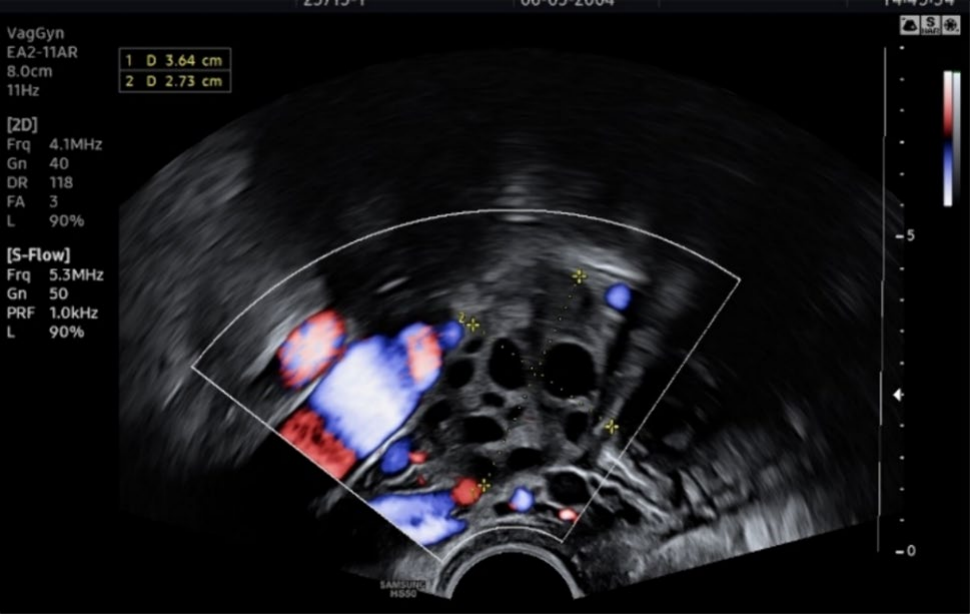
Unremarkable transvaginal sonogram of the left ovary
